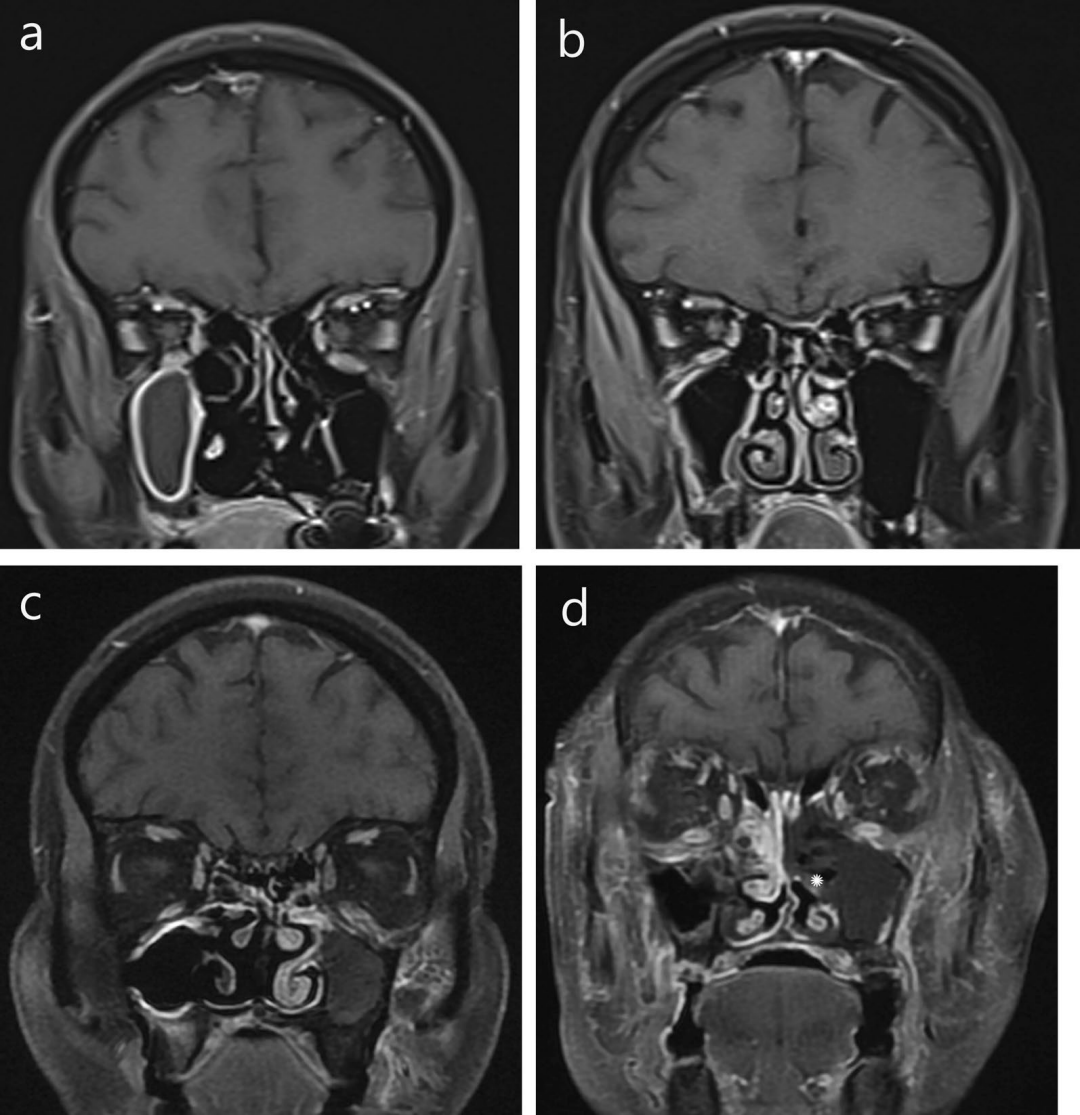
Mucosal enhancement patterns on MRI in mucormycosis. T1 contrast enhanced images in coronal view of face show a intense enhancement, b patchy heterogenous enhancement and c non-enhancing maxillary sinus mucosa, d non-enhancing left middle turbinate—“Black Turbinate sign” (*). Right ethmoidal air cells mucosa show intense enhancement. Extensive inflammation is seen in bilateral extraconal orbital fat, temporal and infra-temporal fossa
